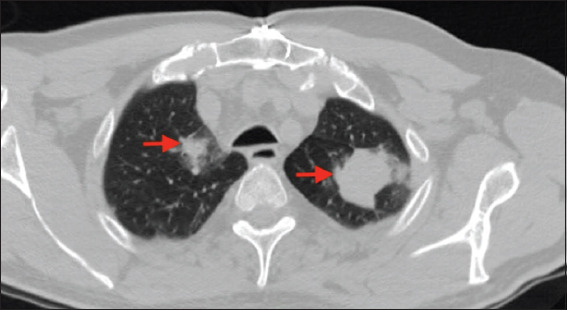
Thoracic computed tomography scan displaying bilateral pulmonary nodules (red arrows)
